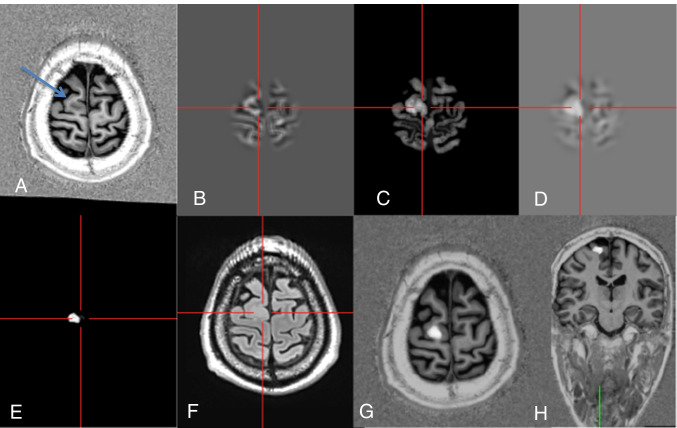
Axial MP2RAGE image with a FCD IIB of the right superior frontal gyrus (A: arrow). Postprocessing with normalization, segmentation, and subtraction/division from a database with 154 healthy controls results in the calculation of junction (B), thickness (C), and extension images (D). These serve among others as input maps for an ANN that creates binary output maps in which the lesion is displayed in gray tones (E). An axial FLAIR image helps to separate the FCD and false positives (F). At the end, co-registered output and MP2RAGE maps are inversely normalized and sent back to the PACS system, in which they are viewed by scrolling through the co-registered data set (G, H) (#12)
